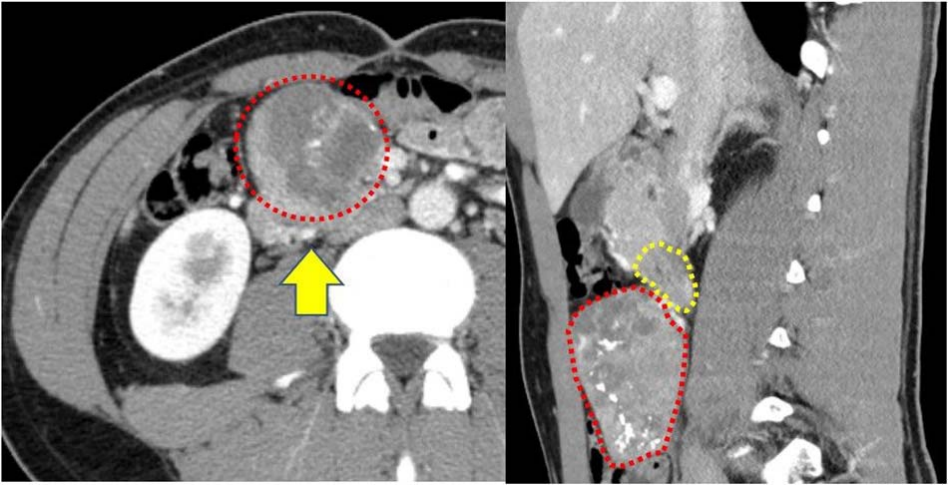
(a) Contrast-enhanced computed tomography reveals a well-defined heterogeneous enhancement mass with calcification (red dotted box); the mass is present on the ventral ileocolic vessels (yellow arrow); (b) the transverse duodenum (yellow dotted box) is pushed by this tumor (red dotted box).
